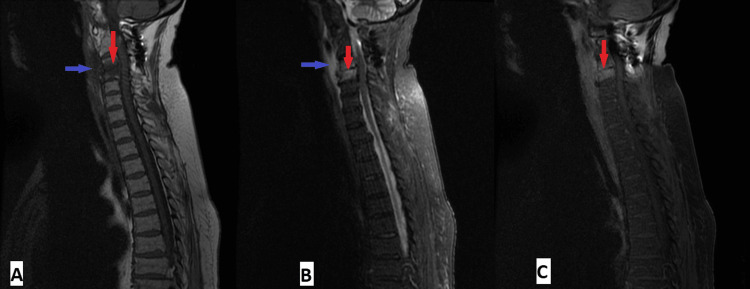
Cervical MRI showing spondylodiscitis. MRI revealed irregularities of the C3-C4 vertebral endplates, accompanied by altered signal from the adjacent bone marrow (vertical red arrows) in both T1 and T2 sequences (A and B, respectively), with enhancement after gadolinium administration (C), reflecting spondylodiscitis in this context, without individualizable lesions/empyema along the vertebral canal, associated with inflammatory signs of the prevertebral muscles, without individualizable abscesses (horizontal, blue arrows).
